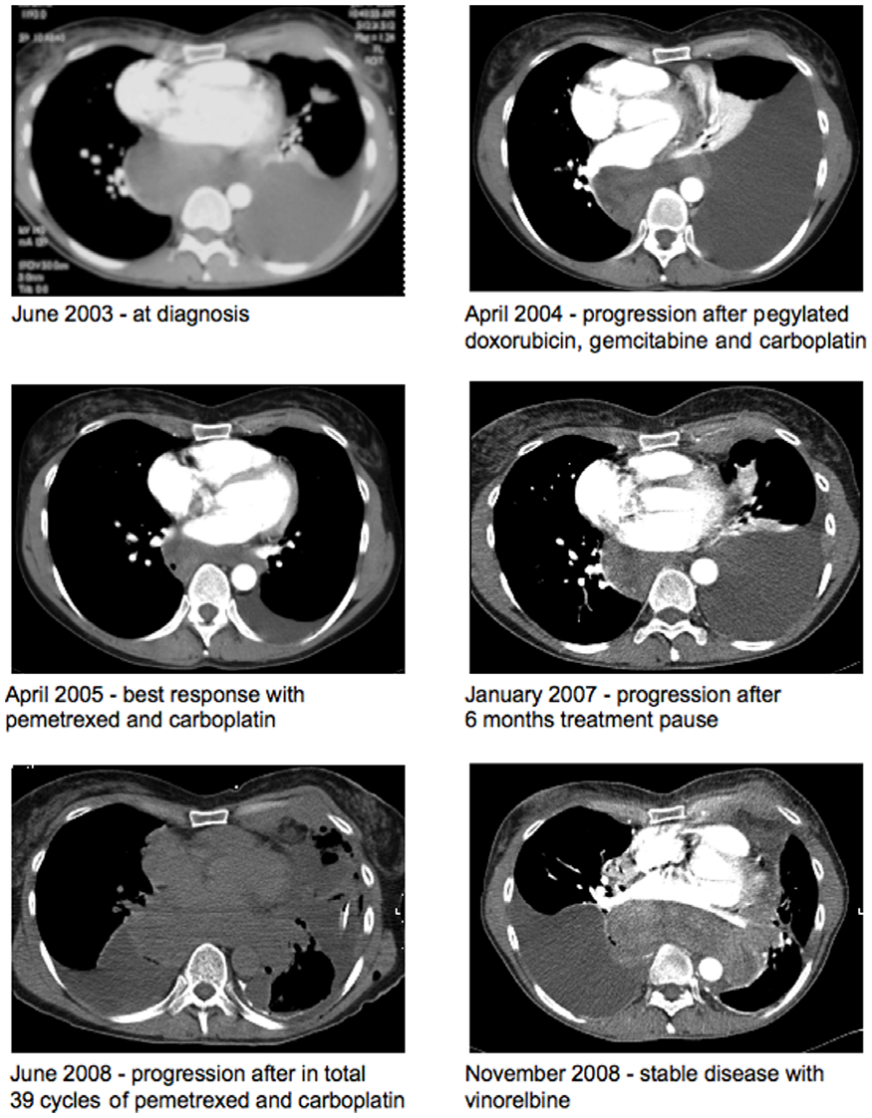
Thoracic computer tomography (CT) throughout the disease course. A large mediastinal tumour as well as thoracic wall infiltration was seen on the left side (CT at the same level). For details see Methods.
